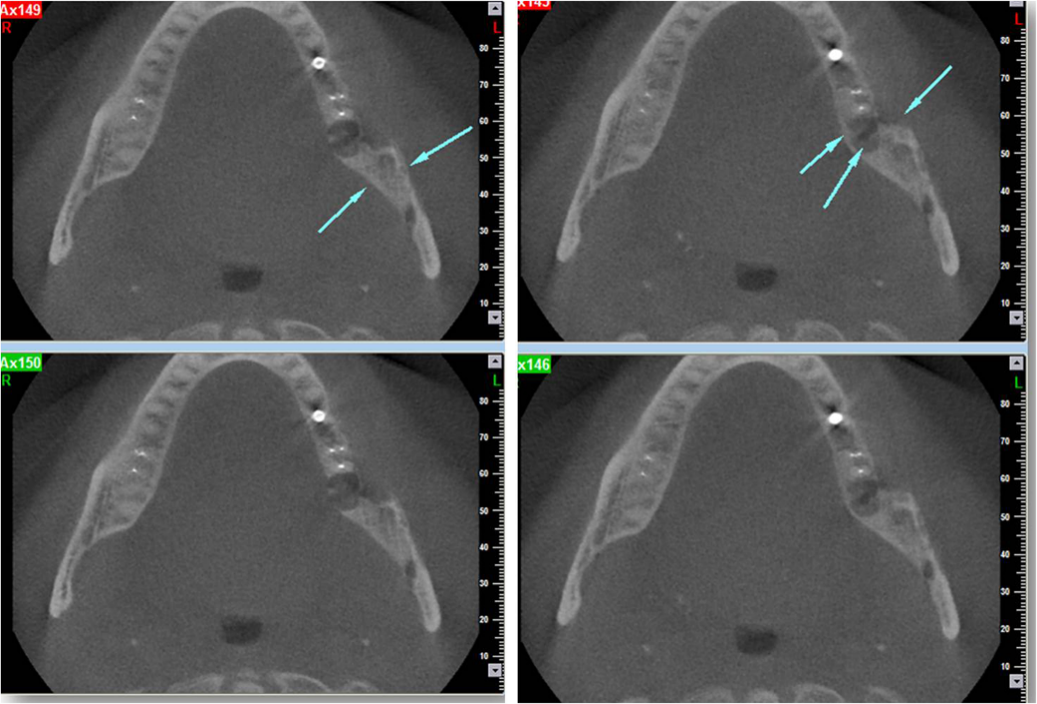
Axial cone beam computed tomography (CBCT) images showing erosion in relation to the mandibular nerve on the level of the lingula mandible.
